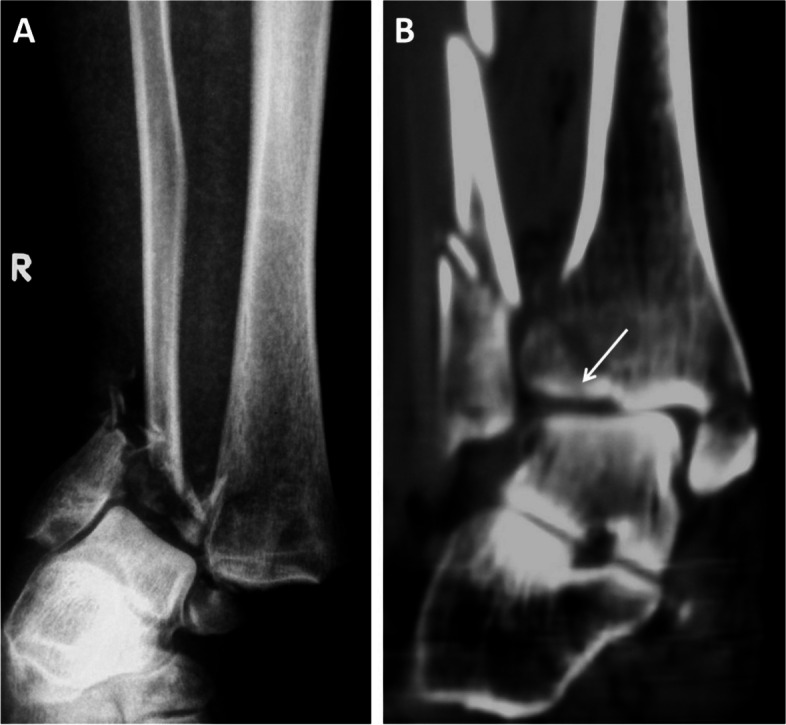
Characteristics of the tibial injury. A The talus was displaced to the syndesmosis, talofibular joint congruence. B Compression fracture of the lateral articular surface of the distal tibia displayed by CT of the right ankle joint (arrow)
